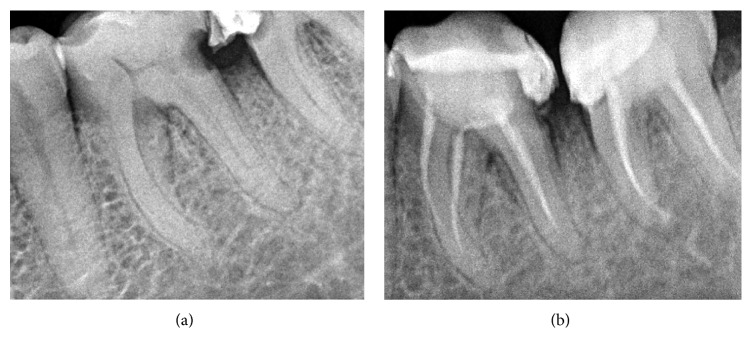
(a) Preoperative radiograph of the left mandibular first molar showing a deep carious lesion on the distal aspect of the crown. (b) Postoperative radiograph of the left mandibular first molar showing an incomplete and incorrect root canal filling, associated with changes of the initial curvature of the mesiobuccal canal.
